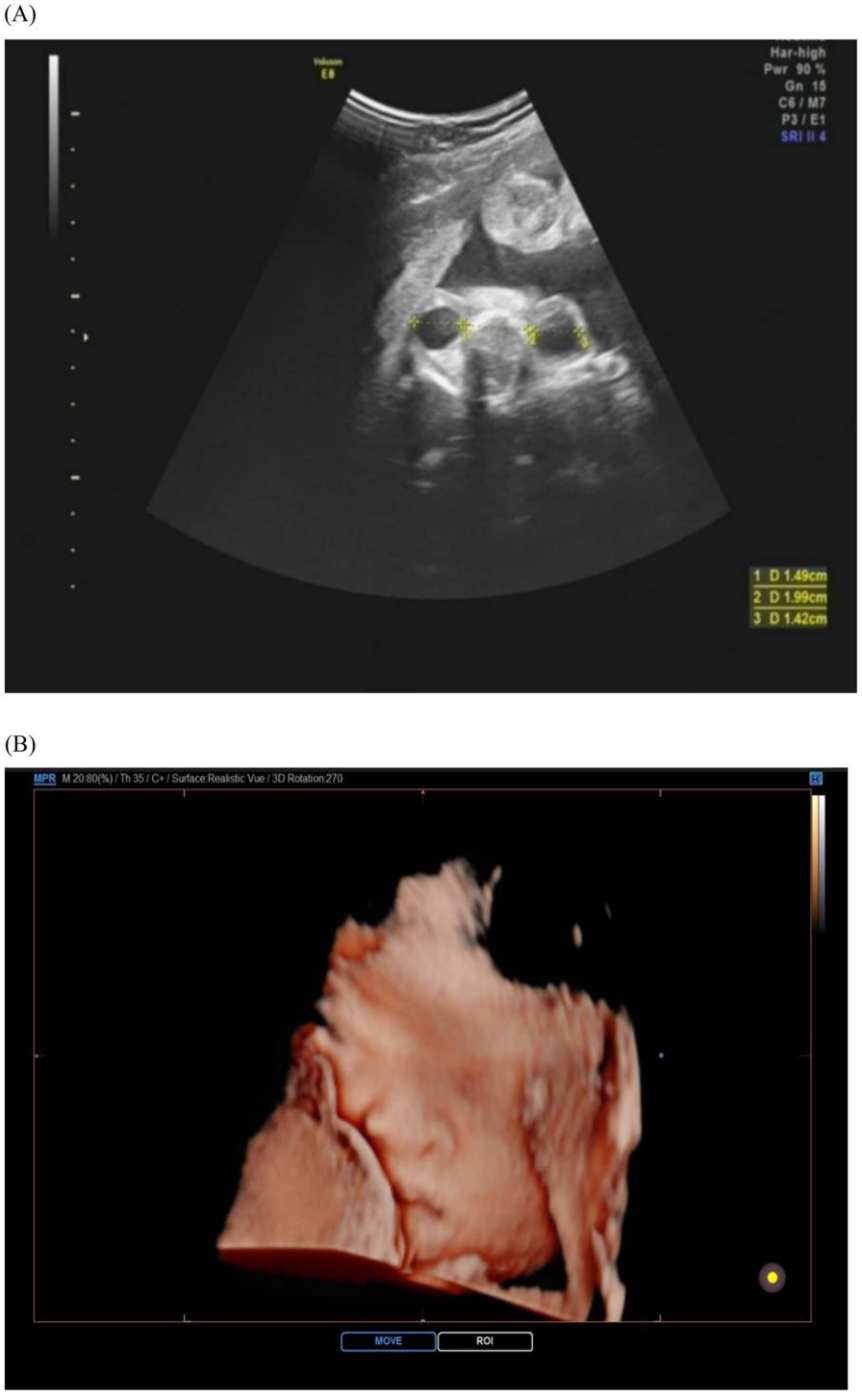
Ultrasound images of fetal face in apert syndrome. The cross-section of the bilateral orbits (A) and the three-dimensional view of the face (B) reveal a depressed nasal bridge and hypertelorism. The orbits appear shallow, resulting in relatively prominent eyes.
